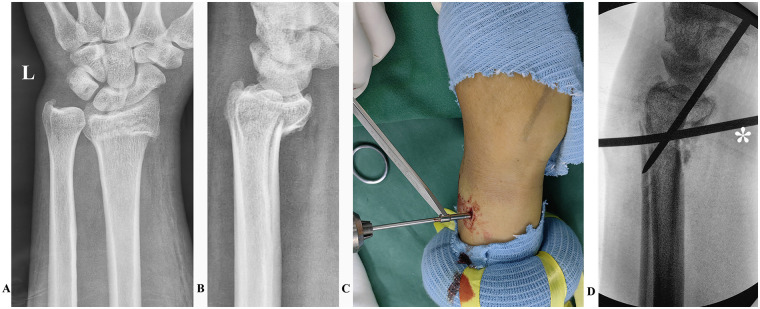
A 51-year-old male patient suffered from depressed distal radius fracture (initial type C2) 4 months before admission. (A) A PA X-ray taken immediately after surgery. (B) A lateral X-ray. (C) Multiple pin holes were made for osteotomy. (D) The distal bone fragment was distracted with a pin (*) to restore the normal length of the radius.
